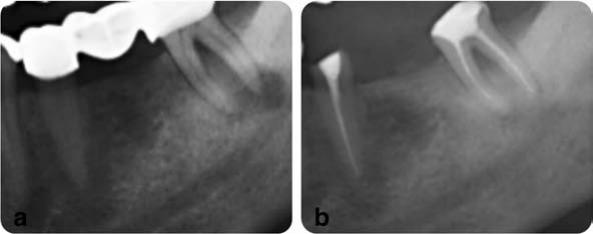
A representative x-ray image of complete healing, a before RCT, b a-year follow-up
